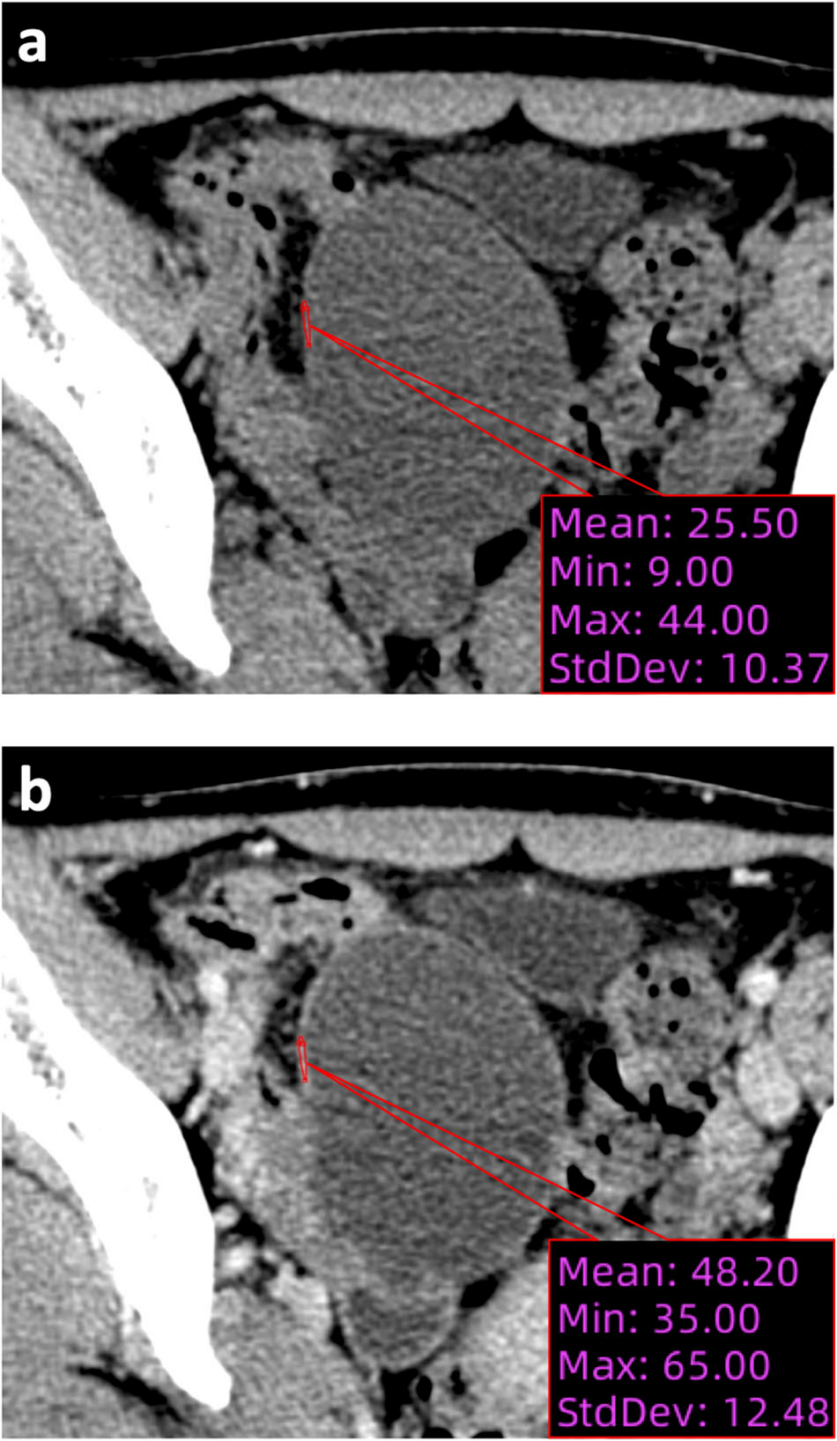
Attenuation measurement in contrast-enhanced CT of a patient (age: 22 years) with a chief complaint of abdominal pain for 4 days. Surgically and pathologically confirmed untwisted ovarian cyst. a Non-contrast phase (attenuation: 25.5 HU). b Portal-venous phase (attenuation: 48.2 HU). The ΔHU_PV-NC_ is 22.7 HU
